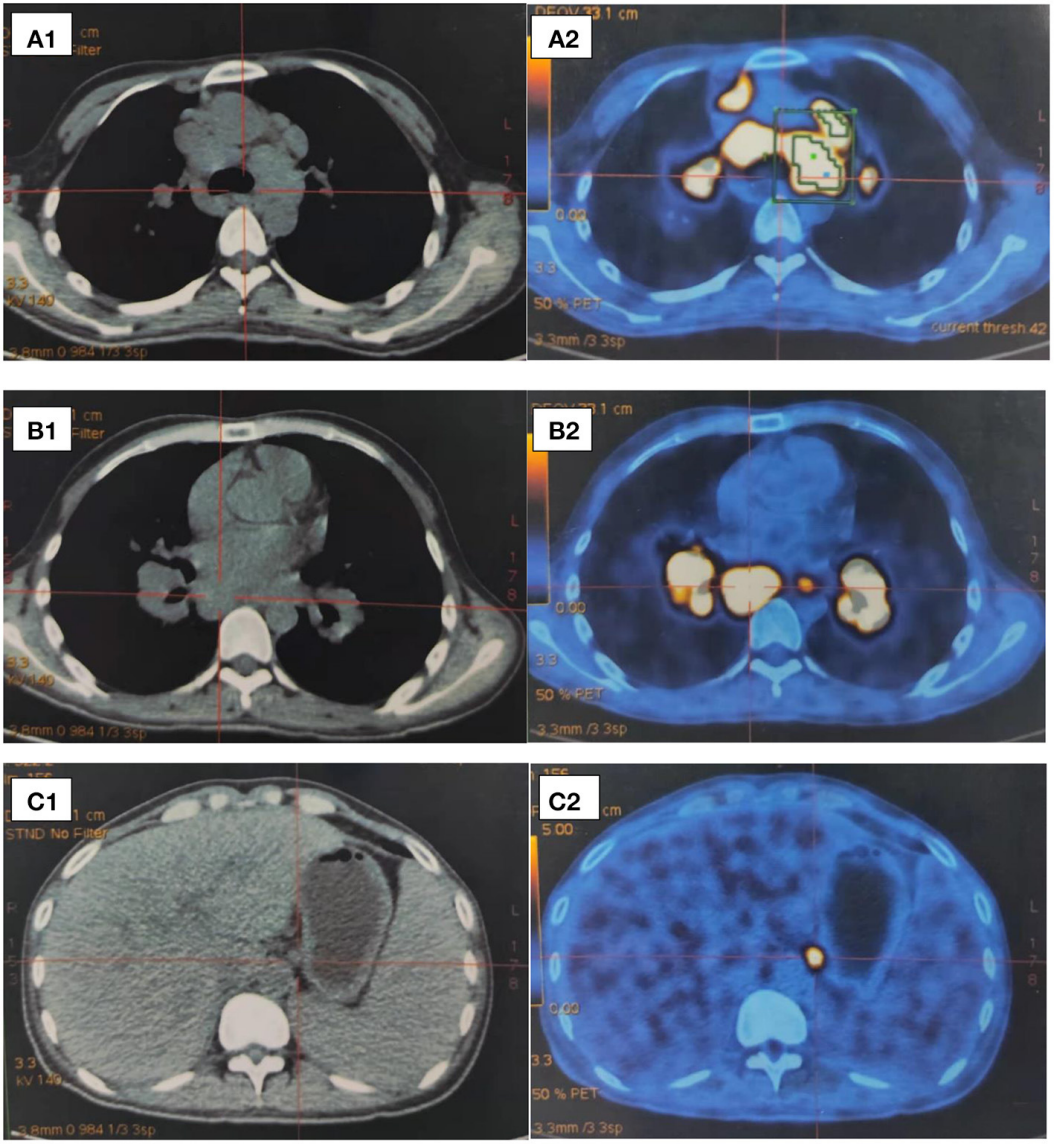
(A–C) Partial images of positron emission tomography-CT scan in 2017. (A) Mediastinal lymph nodes enlargement (tracheal carina) SUV max = 18.39; (aortopulmonary window) SUV max = 18.93. (B) Hilar lymph nodes enlargement. (C) Markedly enlarged spleen.
